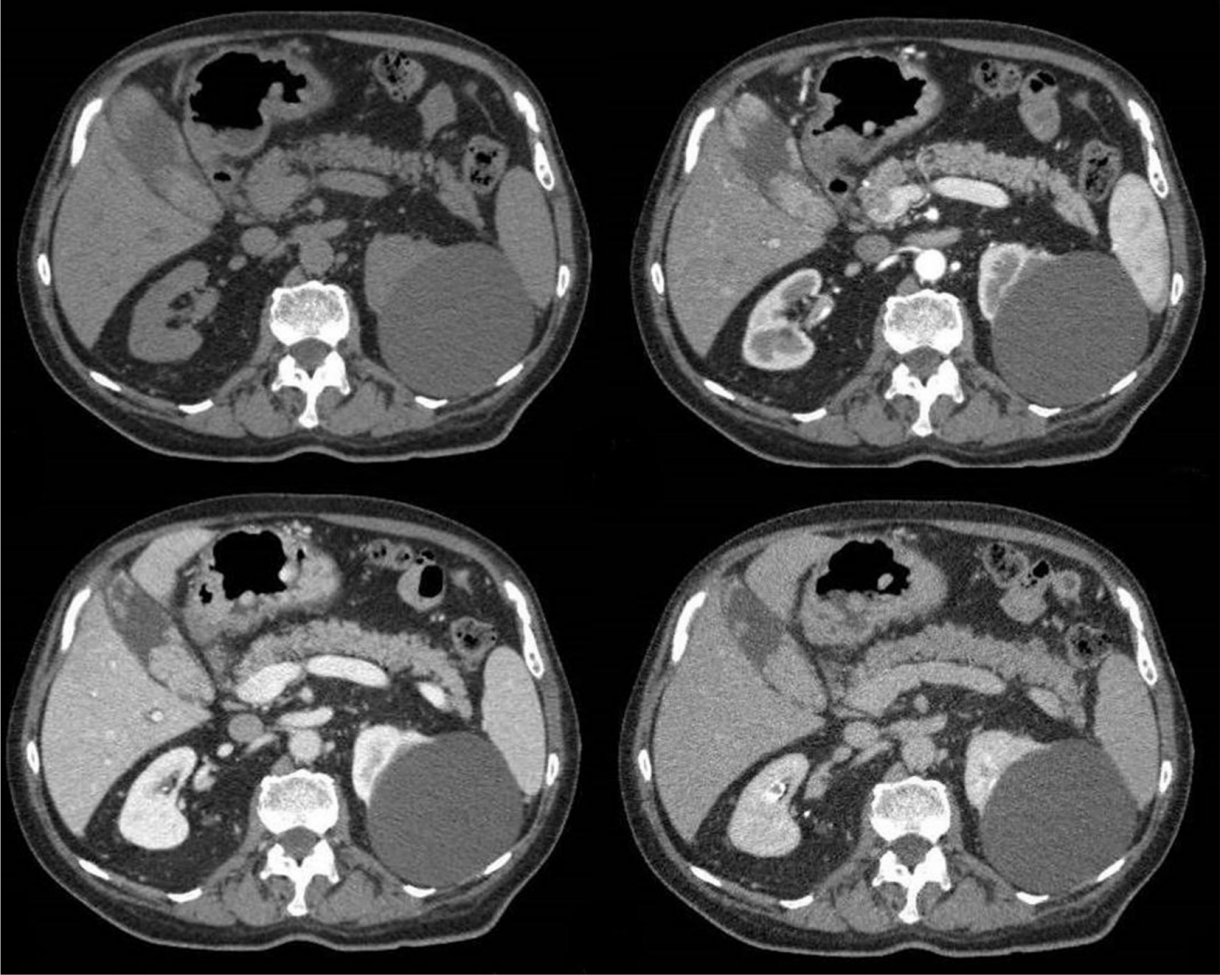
(a–d) CECT, contrast-enhanced CT of the abdomen . Multiple nodular lesions in the gallbladder show early intense enhancement followed by progressive washout .
